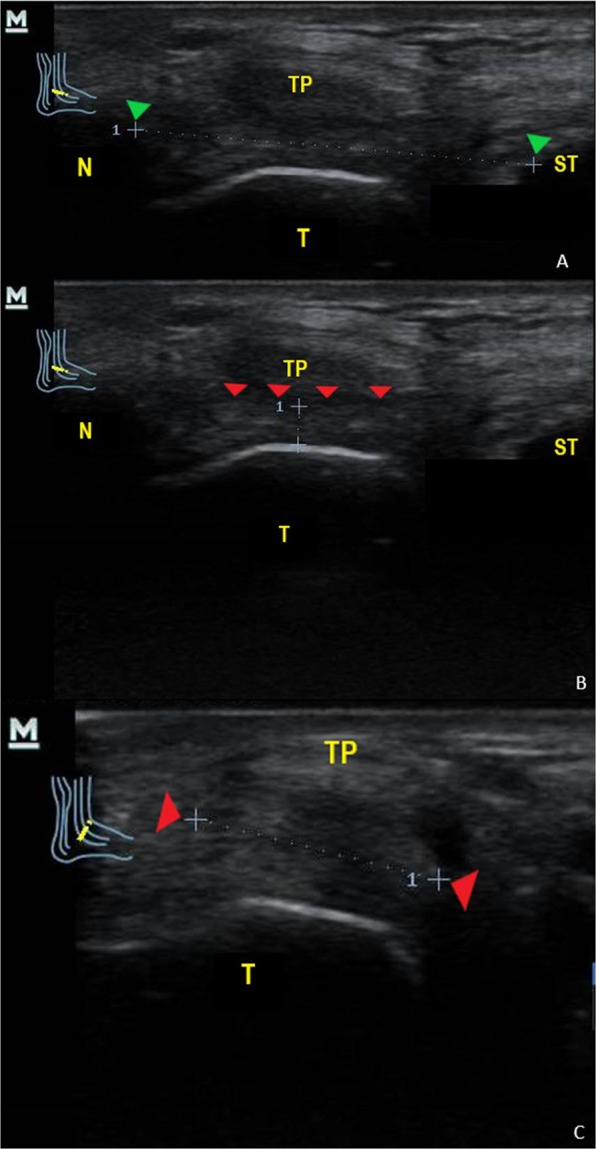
Ultrasound measurements of the Spring Ligament for length (A), thickness (B) and width (C) dimensions in cadaver foot. *Abbreviations:* N, Navicular; ST; Sustentaculum Tali; T, Talus; TP, Tibial Posterior tendon. Green arrows showed bone references of Navicular and Sustentaculum Tali for length measurements. Red arrows showed Spring Ligament references for thickness and width measurements.
